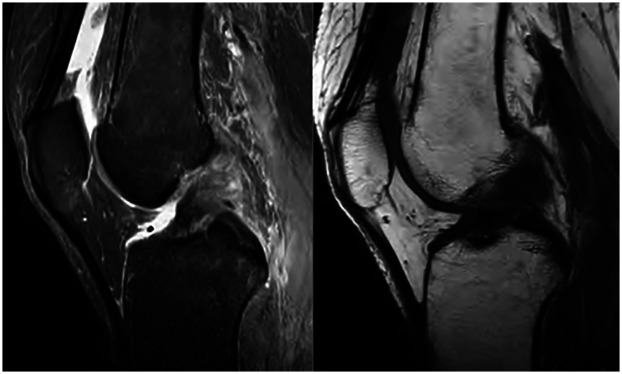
Preoperative MRI images showing anterior cruciate ligament rupture.
